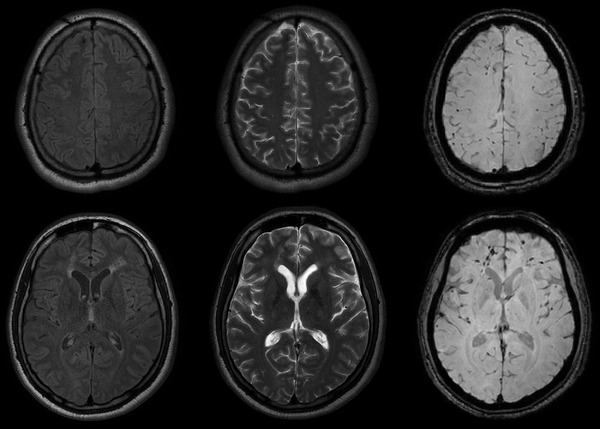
Axial MRI images of a patient evidencing small hemorrhagic lesions characteristics of diffuse axonal injury in the FLAIR (left), T2‐weighted (middle), and PRESTO images (right)
